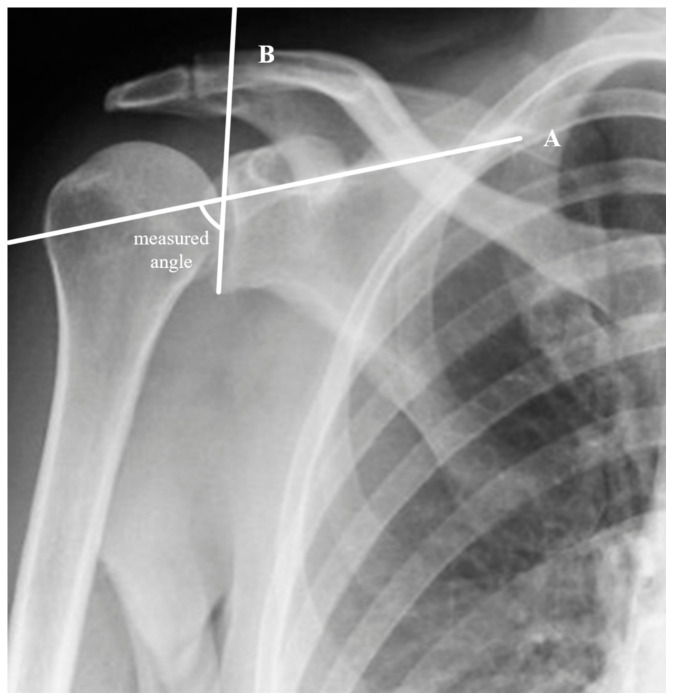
Anteroposterior shoulder radiograph demonstrating the measurement of the Glenoid inclination. Three anatomical reference points are identified: the most superior point of the glenoid; the most inferior point of the glenoid and the supraspinatus fossa. Glenoid inclination was calculated using the angle between the line (B) drawn along the supraspinatus fossa and the line (A) connecting the most superior and inferior points of the glenoid on the anterior–posterior shoulder radiograph. The inclination value was determined as the value obtained by subtracting the measured angle from 90°.
